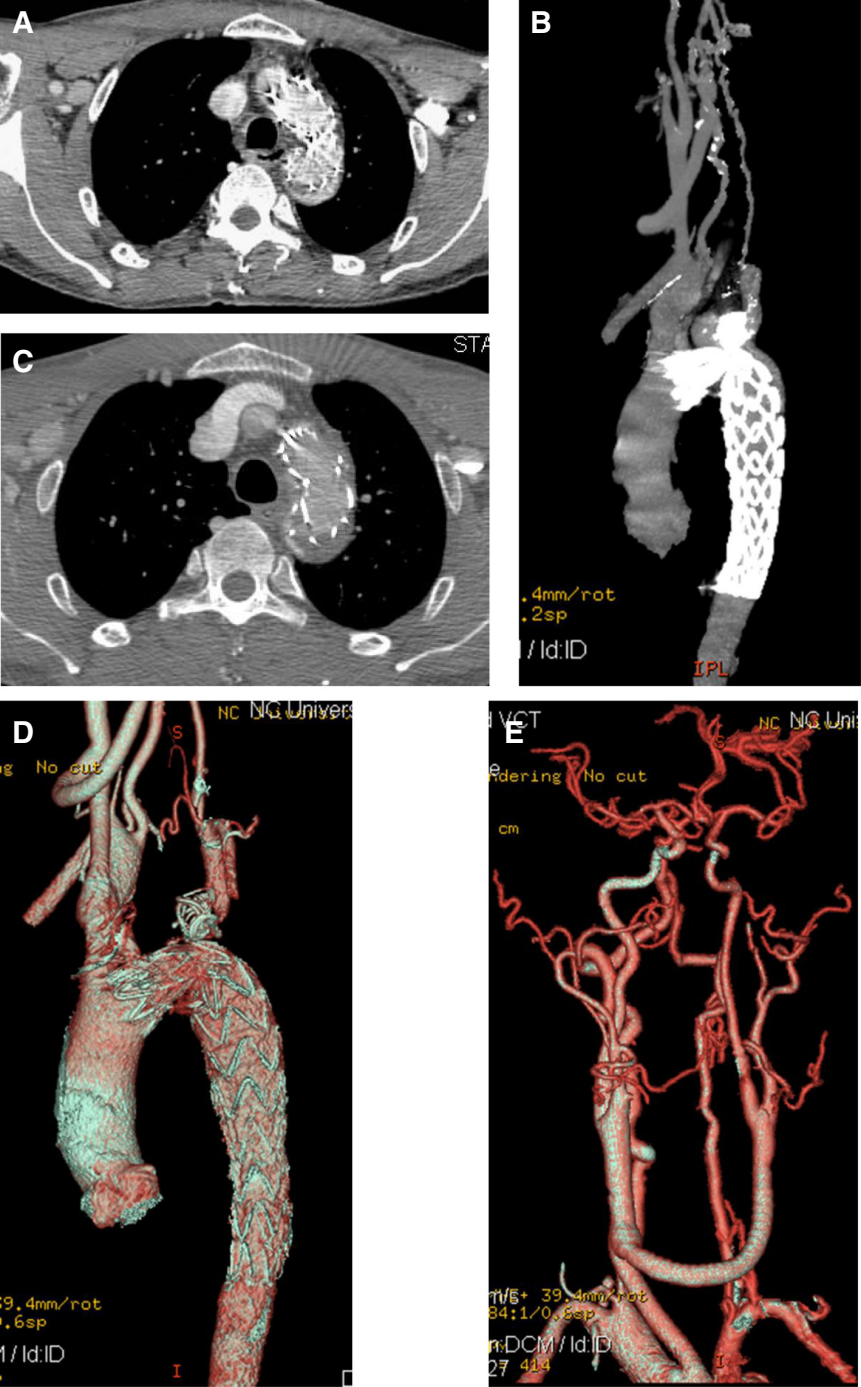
CTA demonstrated aortic arch aneurysm sac with thrombosis and a small endoleak, and patent carotid to carotid bypass in one week follow-up (A, B). Postoperative CTA demonstrated thrombosis in the aneurysm lumen, no endoleak and the aortic arch and the carotid to carotid bypass were all patent except very mild stenosis of LICA at 8-month follow-up (C, D, E).
